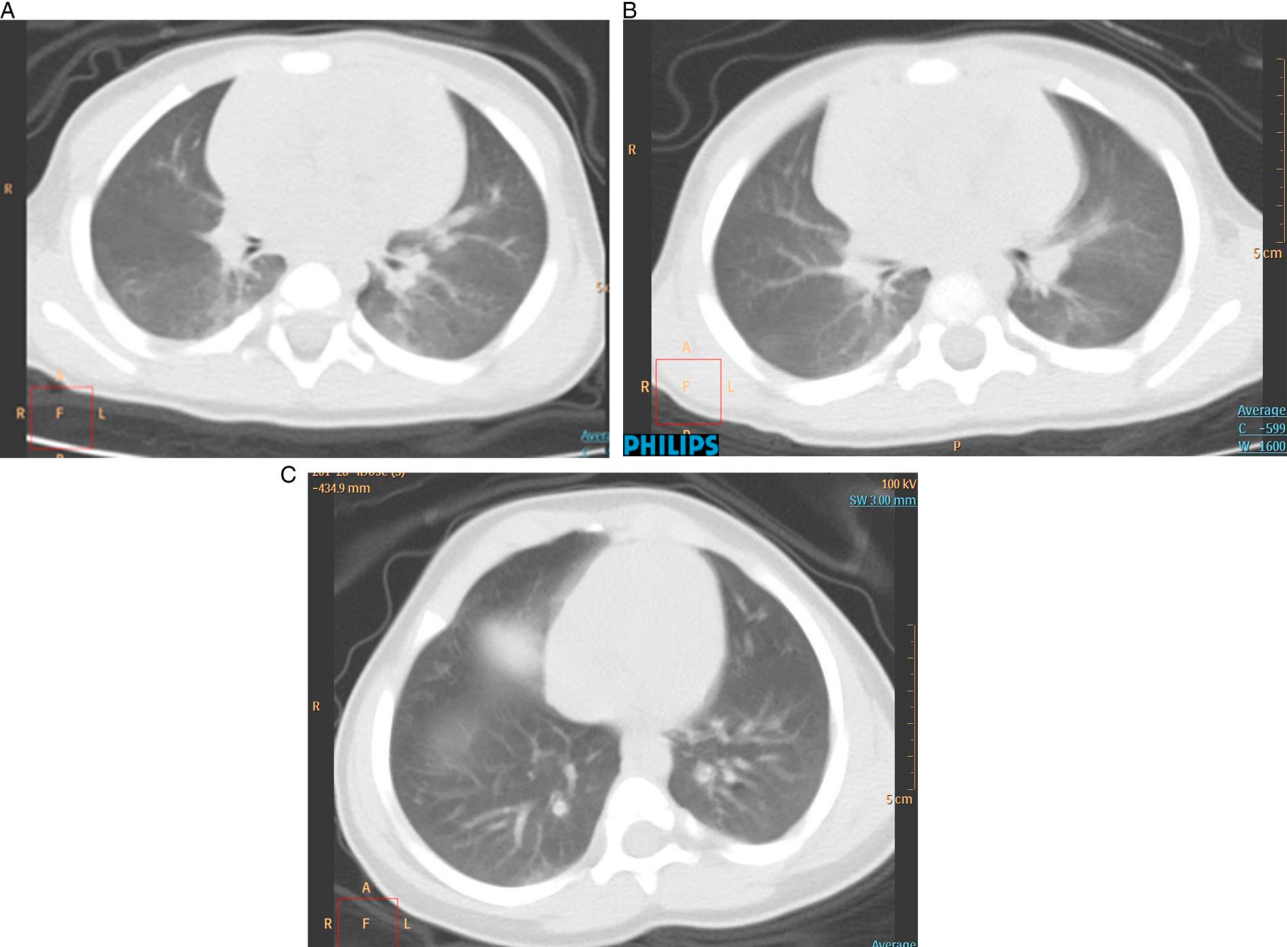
Chest CT images of a case 7 and case 8 (one pair of 11 month old twins) patient upon admission, who had no symptom. (A) Transverse chest CT images showed pulmonary consolidation and ground glass opacity (case 7 on 7 February). (B) Showed marked improvement after 5 days (case 7 on 13 February). (C) Lung CT of case 8 – the case 7’s younger brother on admission day (case 8 on 7 February).
